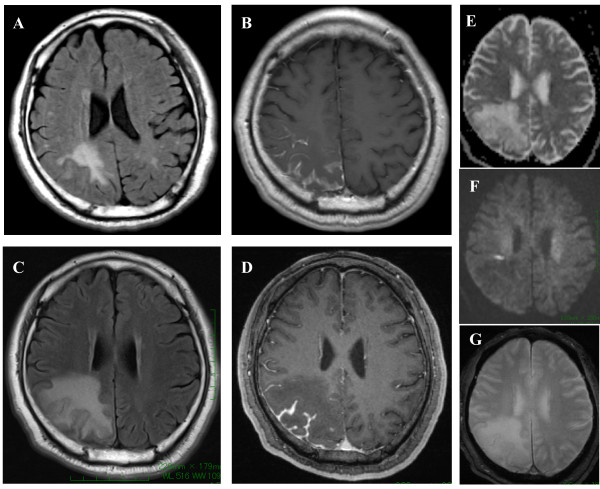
Axial MRI from the referring hospital and on admission to our hospital. MRI findings of FLAIR (A) and T1-weighted image with Gd enhancement (B) from the referring hospital (1.5T). Increased white matter lesions are visible in the right parietal lobe on FLAIR images (A), and a T1-weighted Gd enhanced image revealed abnormal enhanced parenchymal lesions along the parietal sulci (B). On admission, these lesions worsened in both FLAIR (C) and T1-weighted enhanced images (D). High signal intensity in the apparent diffusion coefficient (ADC) map (E) and low signal intensity in the diffusion-weighted image (F) suggested its edematous nature. No microhemorrhages were observed with Gradient recalled echo-T2* imaging (3T) (G).
